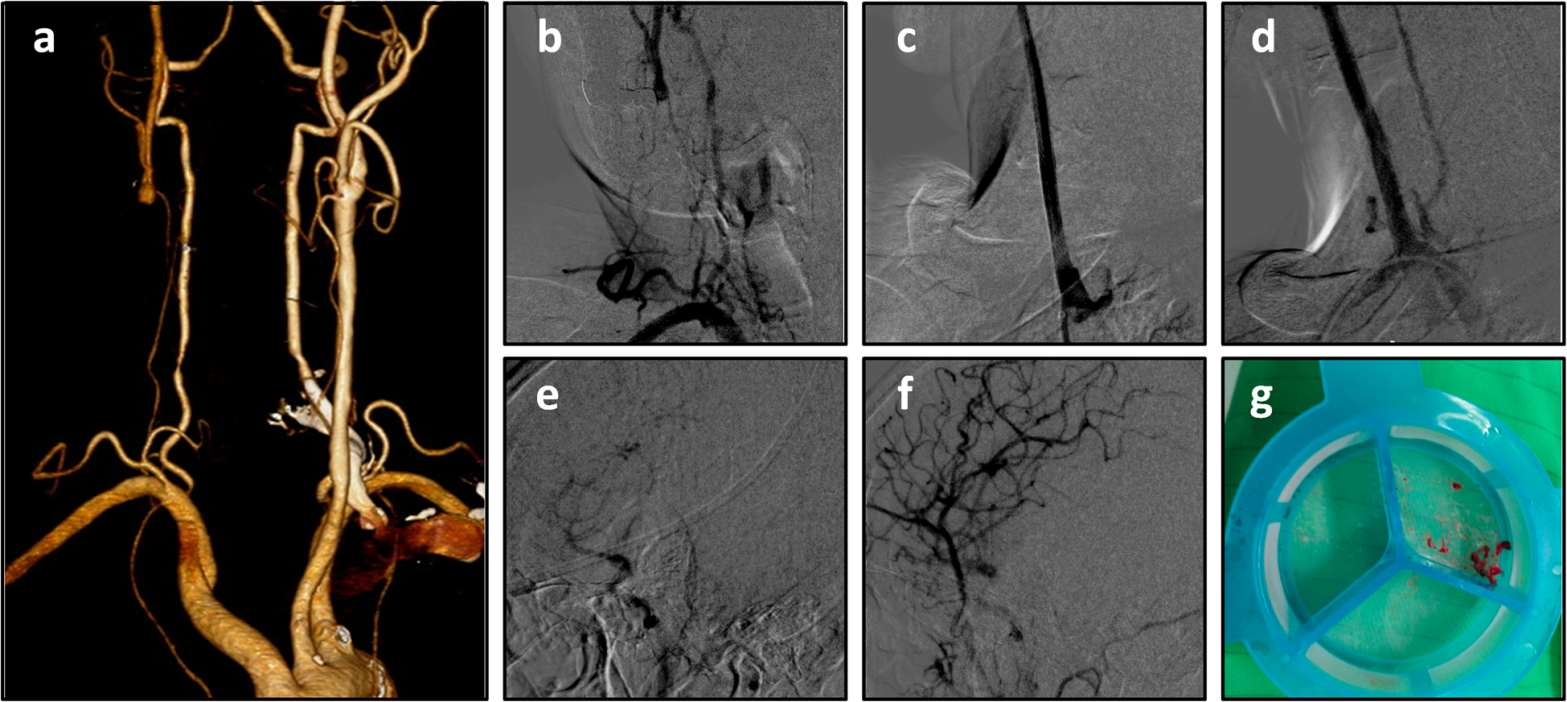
Patient 1 computed tomography angiography: right common carotid artery total occlusion (a). Angiography (b). Final result of angiography (c). Angiography 3 months later (d). Intracranial angiography before intervention (e). Intracranial angiography after the intervention (f). Thrombus (g)
